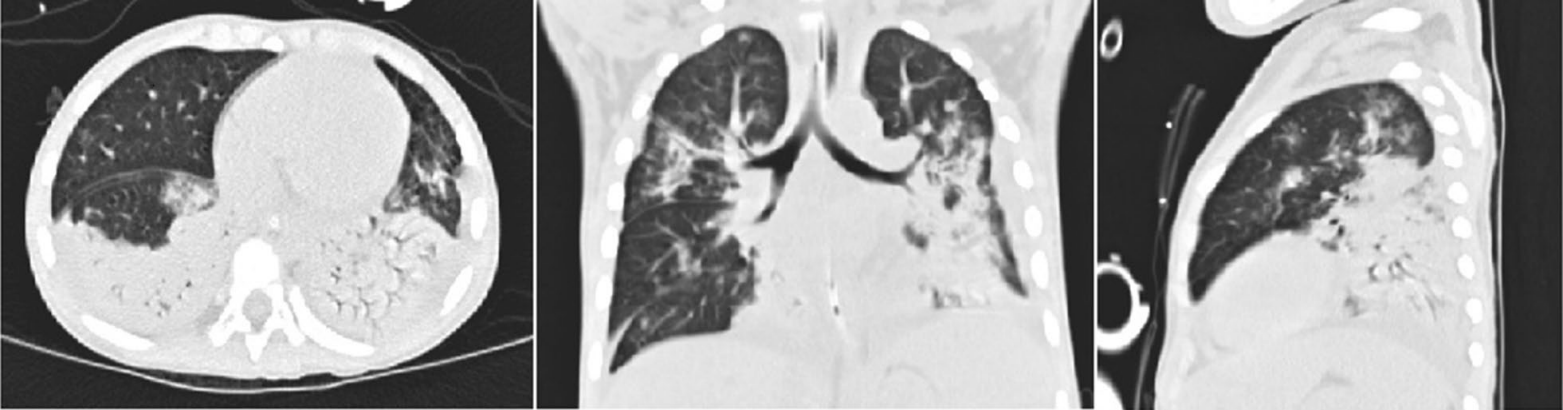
High-resolution CT scan of the chest revealing areas of airspace consolidation in bilateral lower lobes in a 3-year-old child with severe adenovirus (*Adv.*) pneumonia
